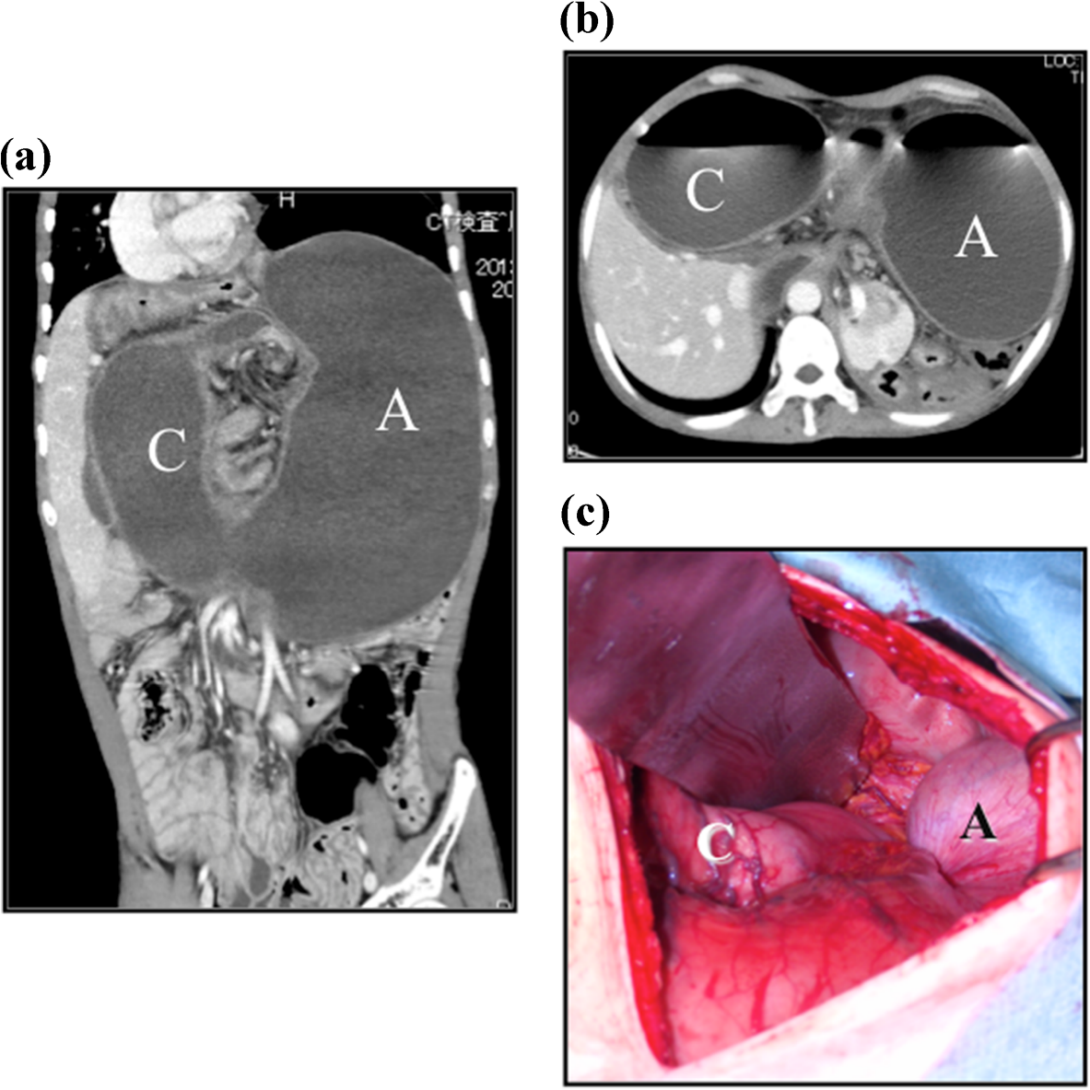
Preoperative and operative findings. Axial (a) and coronal view (b) of the enhanced computed tomography revealed dilatation and volvulus of the stomach. Laparotomy (c) showed a distended stomach rotated 180° around mesoaxis at the cardia and pylorus. A, antrum; C, cardia.
